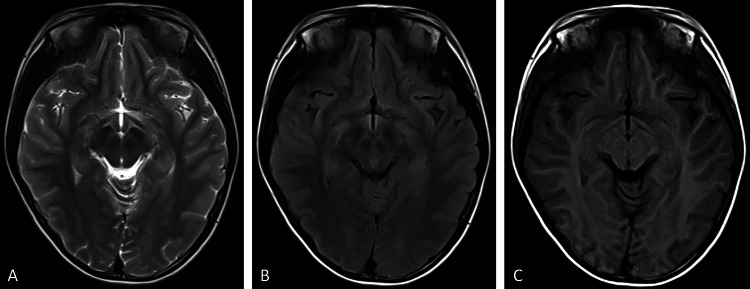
Re-scan imagings of Case 4 Artifact resolution in (A) T2, (B) T2FLAIR and, (C) T1 sequences. FLAIR: Fluid-attenuated inversion recovery.
